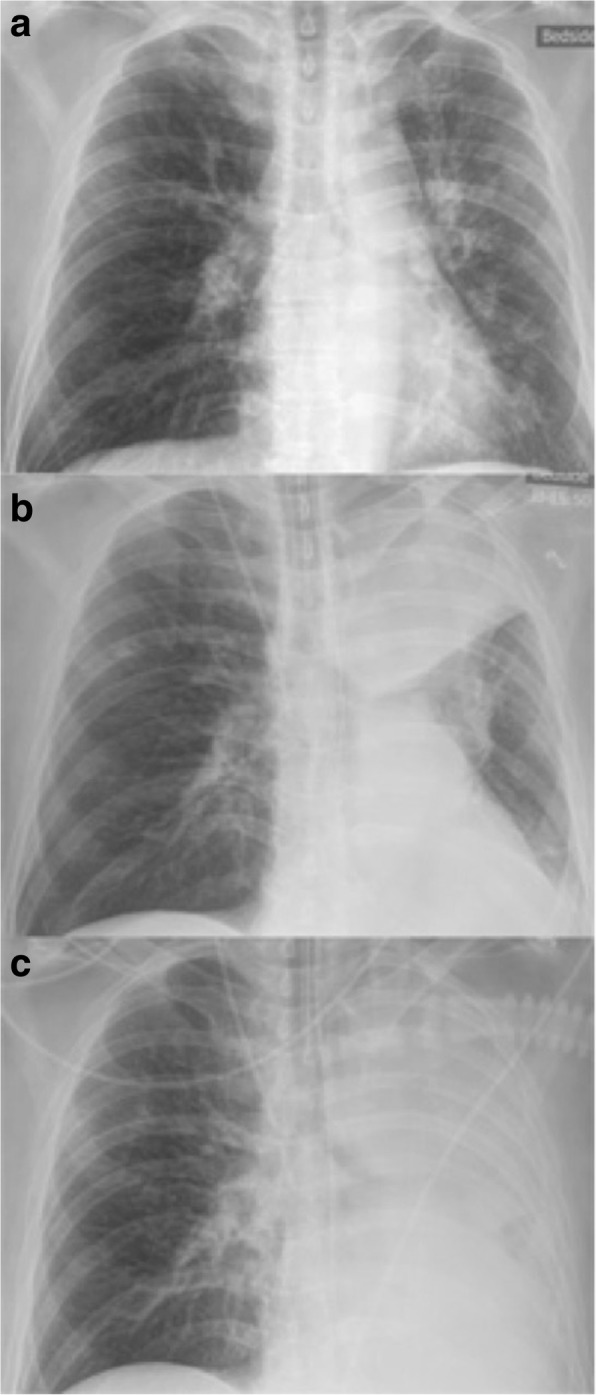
Serial chest radiographs during the first day showing a progressive atelectasis of the left lung. a. Taken at 10.30 in the morning (b). 16.00 in the afternoon and (c). 23.50 at night
